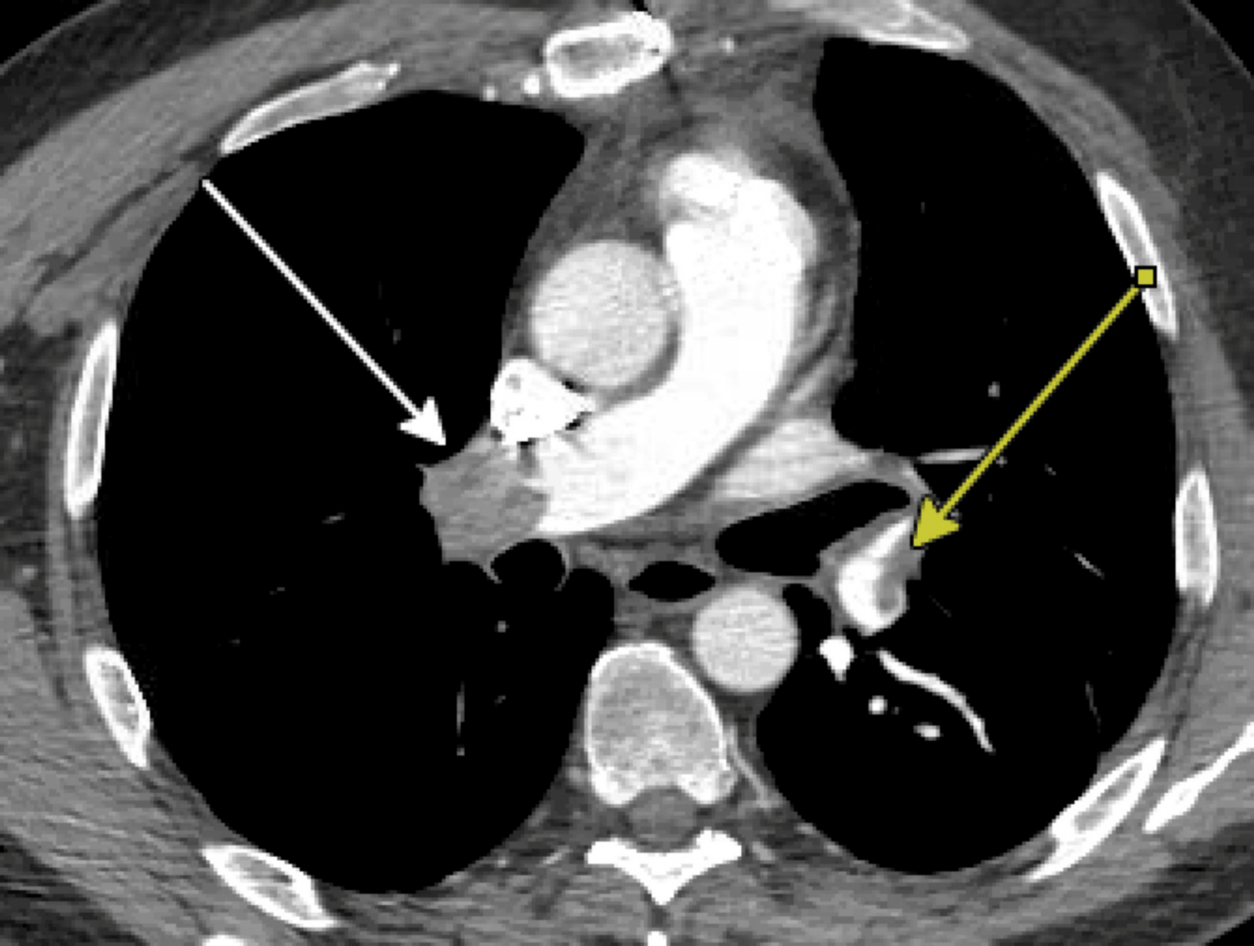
Case 2: CT angiography (CTA) pulmonary embolism (PE) revealing bilateral pulmonary embolisms Bilateral pulmonary embolisms marked with arrows
